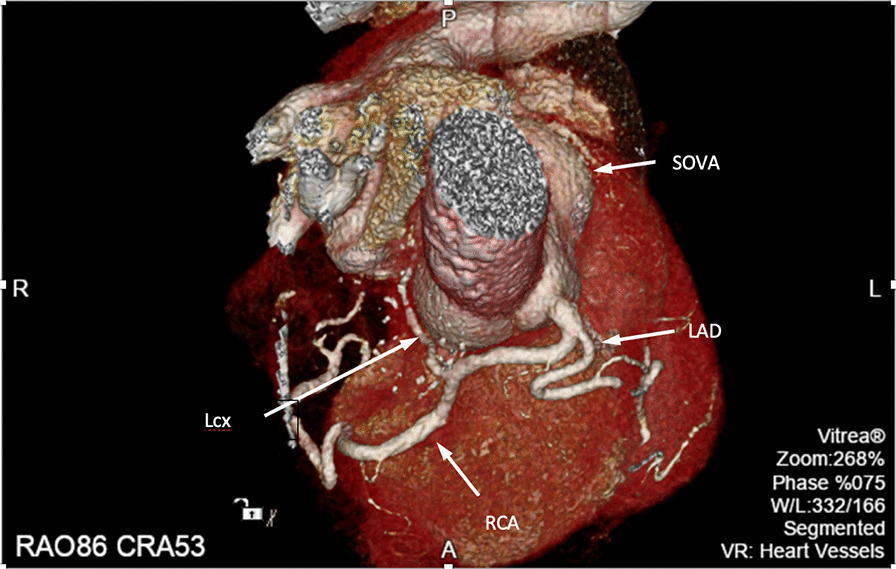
Cardiac CT 3D-rendered images showing the left Sinus of valsalva aneurysm (SOV), the left main origin from the right cusp and left anterior descending artery (LAD) coursing anteriorly while the left circumflex (Lcx) appears to originate from the proximal right coronary artery (RCA) and runs posterior to the aorta. The RCA is seen following its usual course
